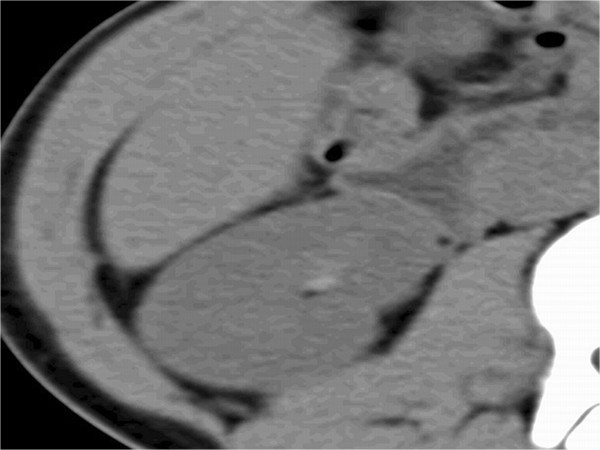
Transverse CT image of 12-year-old patient with phlegmonous appendicitis. No change can be observed in RPS.
